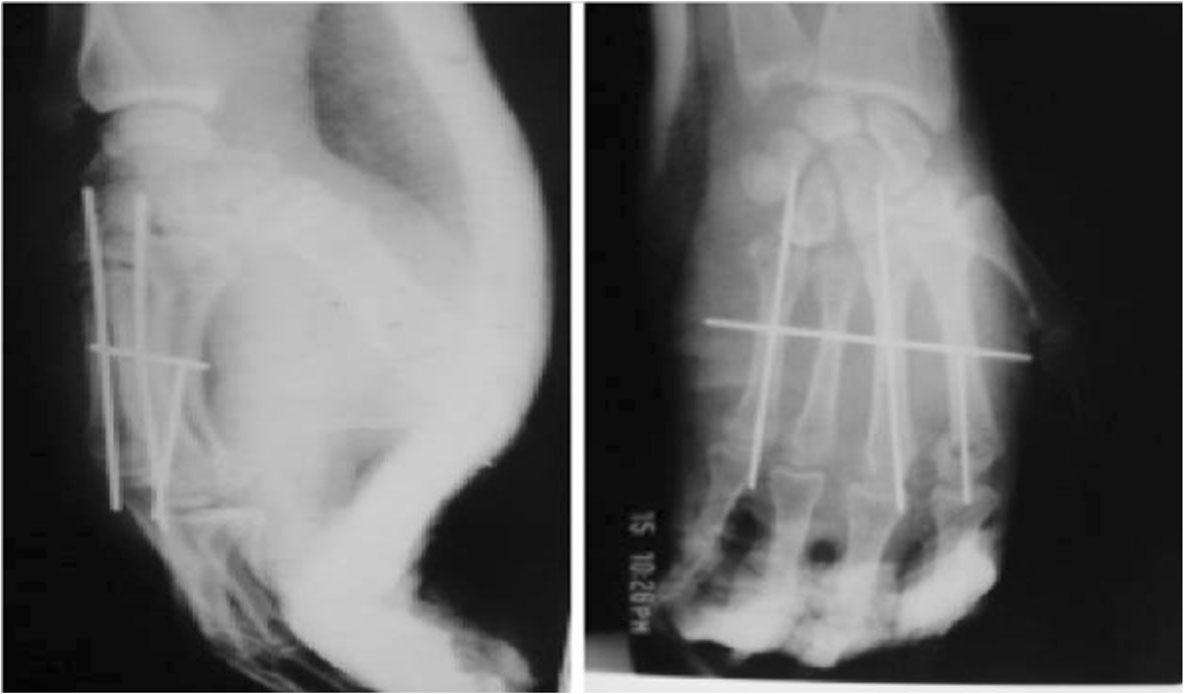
Postoperative radiograph after open reduction and stabilization by an axial pinning of the second, third, and fifth carpometacarpal joints under fluoroscopic control
